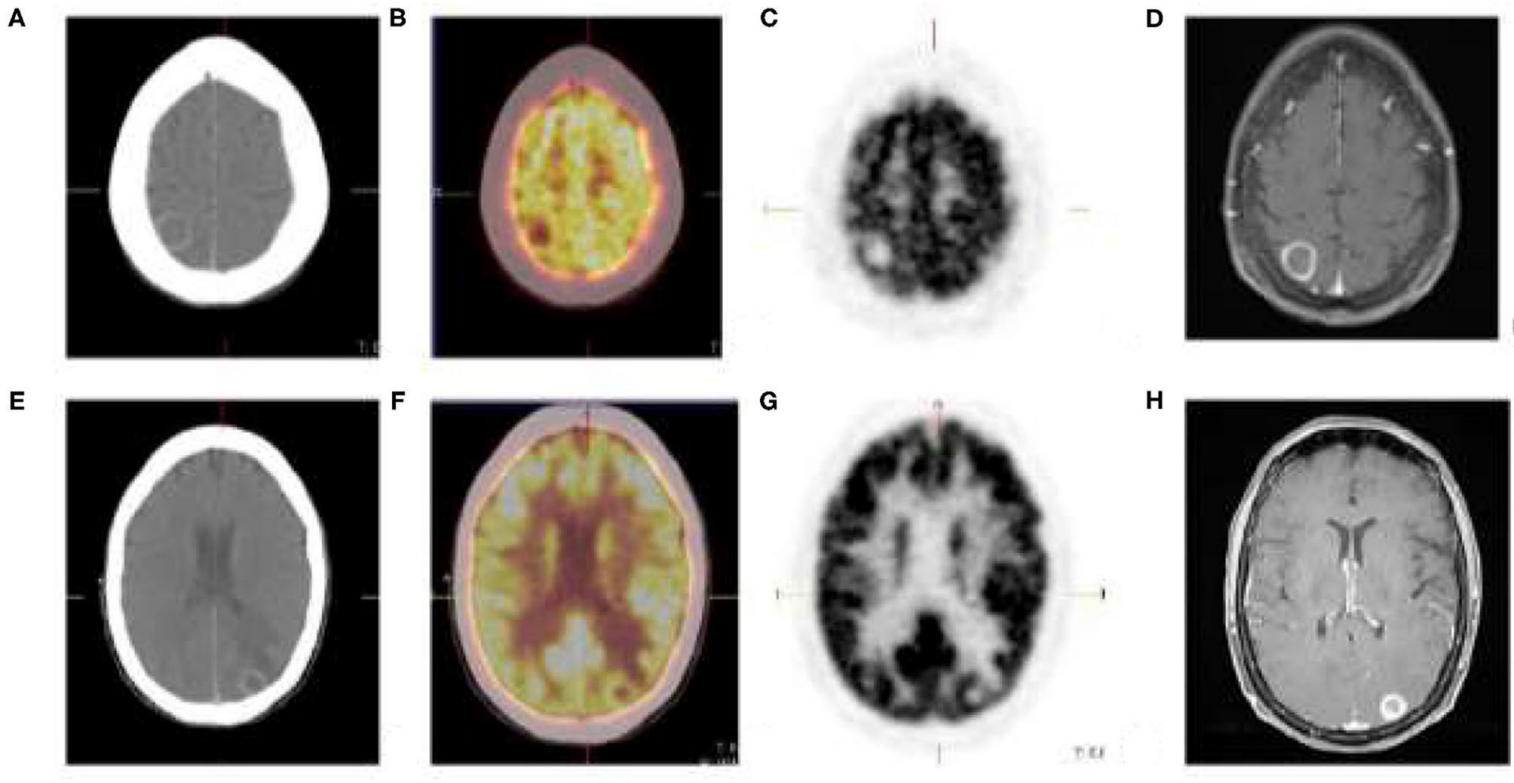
Cutaneous melanoma of the right thigh with known inguinal nodal metastases. [18F]FDG PET/CT shows rim enhancing parietal (A) and occipital (E) brain lesions with no discernable metabolic activity (B,C,F,G). Brain metastases was confirmed on MRI (D,H).
